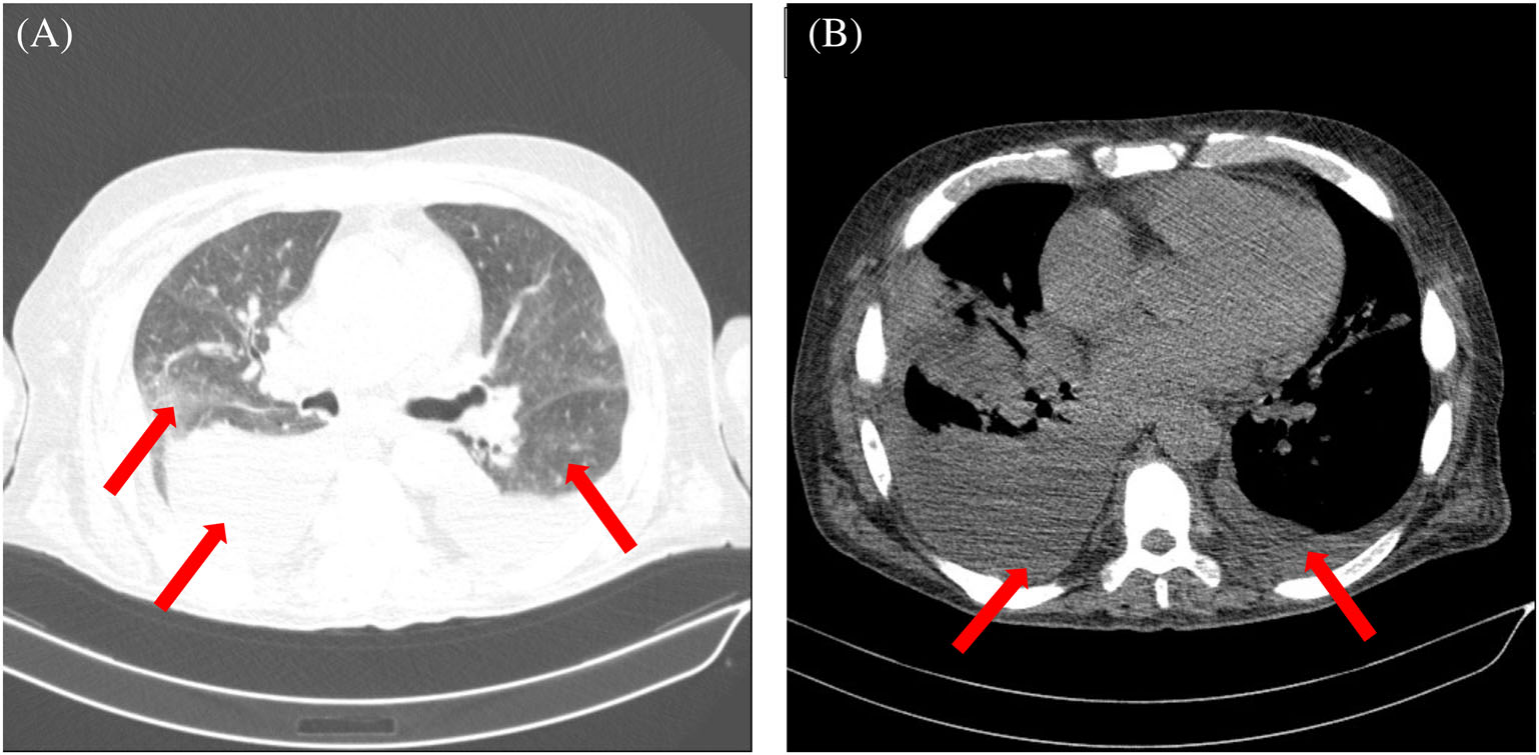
(A) Axial chest computed tomography (CT) scan obtained at pulmonary window of lower region of the thorax showing ground glass opacity and collapse consolidation (arrows). (B) Axial chest CT scan obtained at thoracic window of lower region of thorax showing pleural effusion (arrows).
